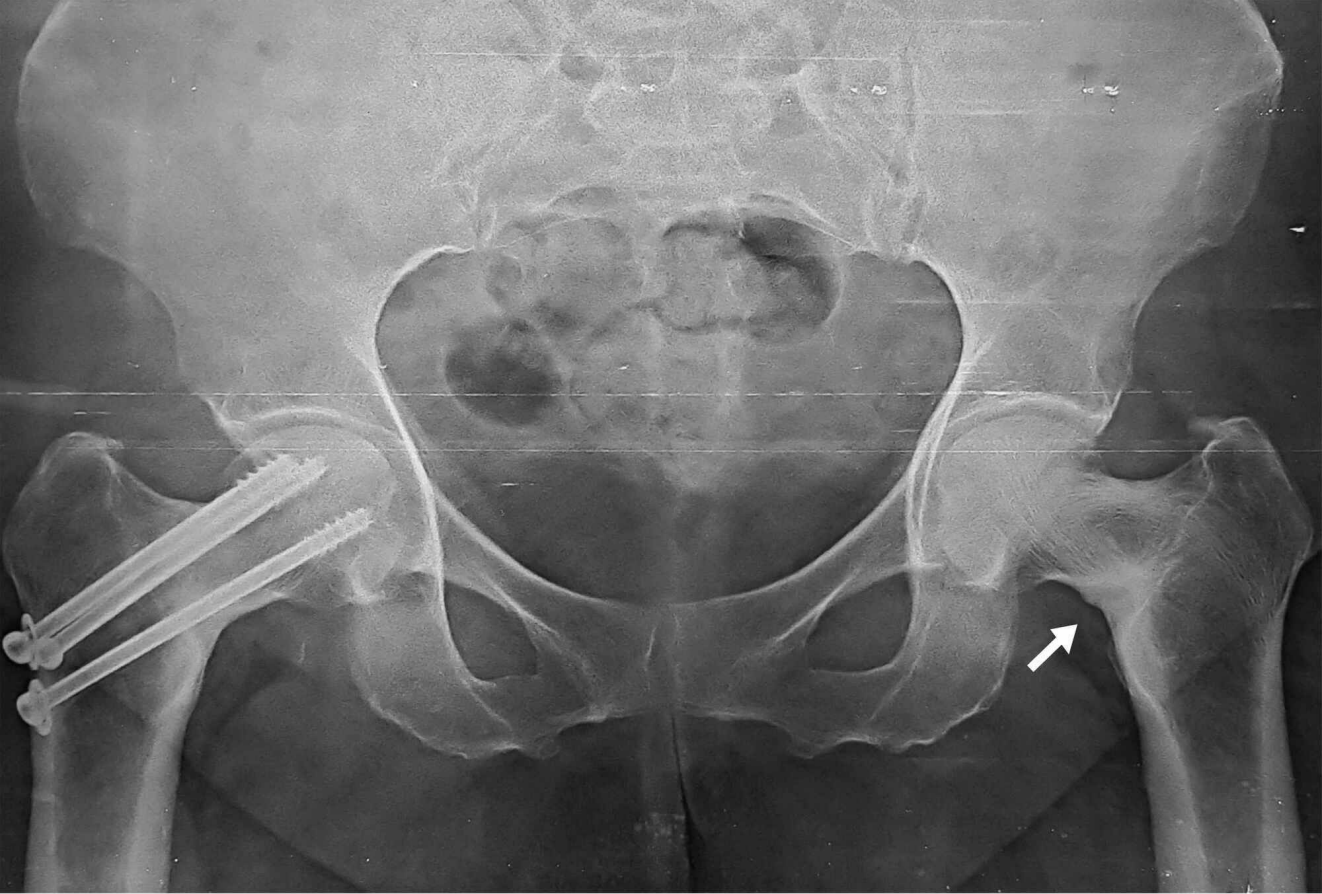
Anteroposterior both hips radiograph with increased sclerosis over bilateral inferomedial femoral neck with no fracture (white arrow).
